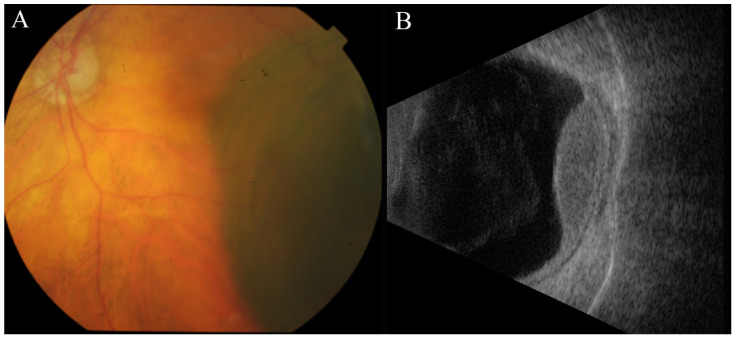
Imaging tests performed on the LE of patient BUM3, compatible with a diagnosis of BUM. (A) Fundus of the LE of patient BUM3 showing a choroidal pigmented mass in the temporal periphery. (B) Ocular ultrasound of LE showing a choroidal mass compatible with a UM measuring 4.5 mm in height and 15.3 mm in base.
